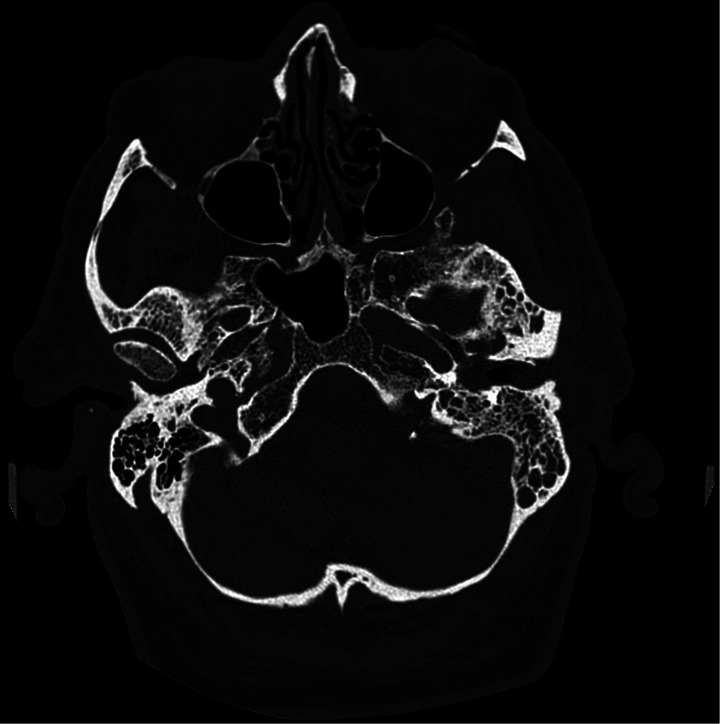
CT scan showing mastoiditis afflicting left ear
